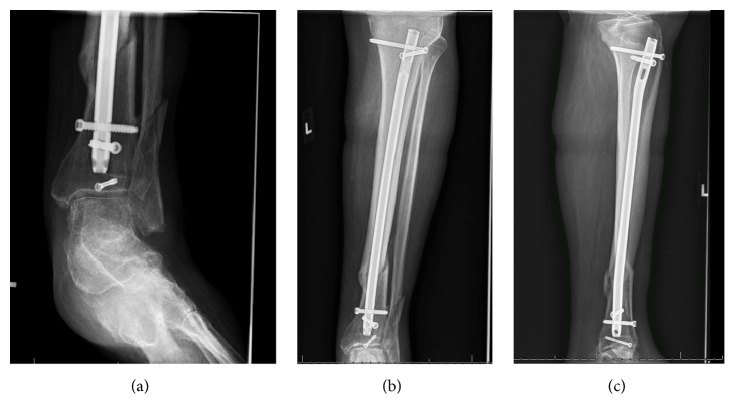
Radiographs at 2-month follow-up showing interval healing with callus formation.
